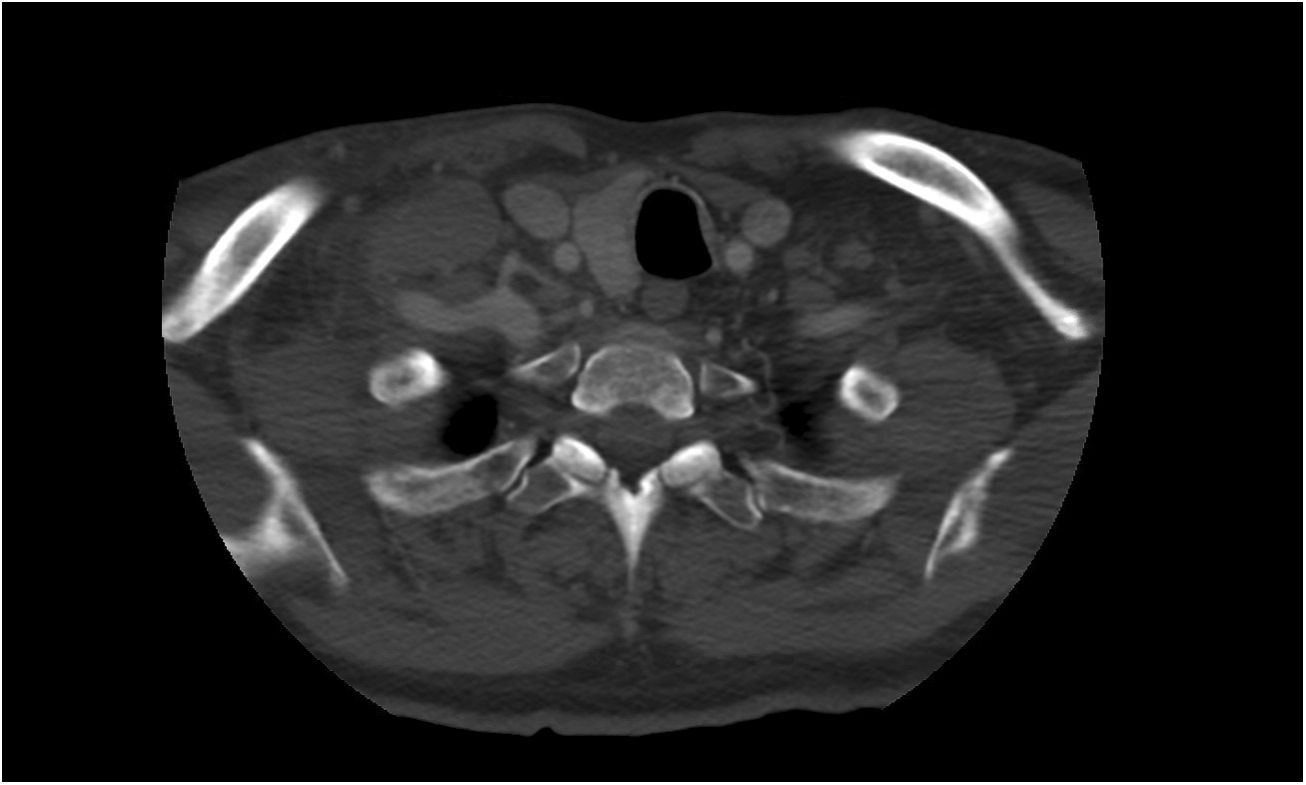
CT soft tissue neck with contrast (coronal view) highlighting large right sided supraclavicular lymphadenopathy.
